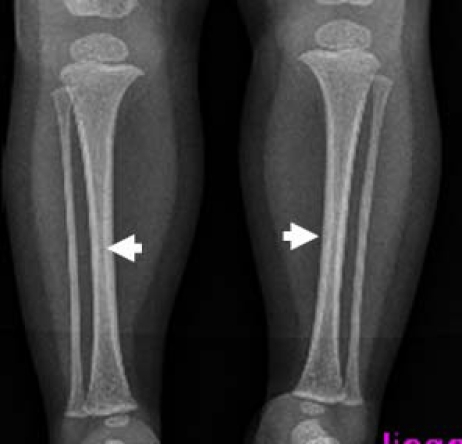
Anteroposterior lower limb radiograph showed relatively broad bones associated with mid-diaphyseal endosteal thickening and medullary narrowing of the tibiae and fibulae respectively (arrows).
